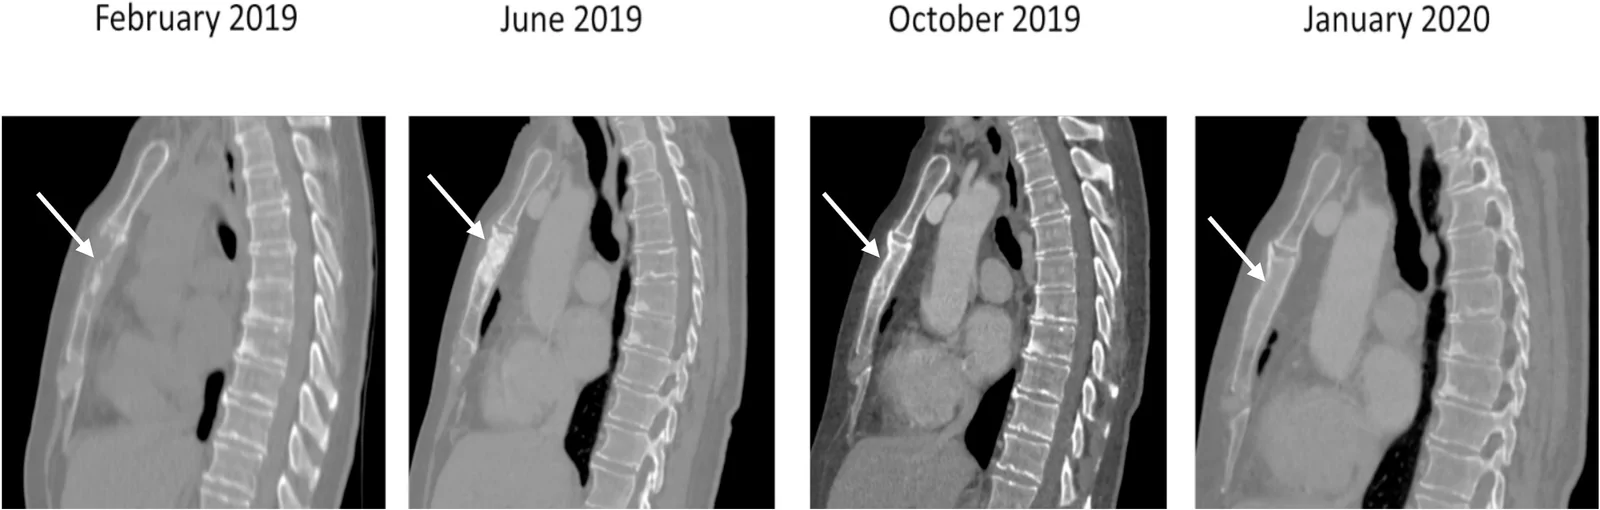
Tumor response of sternal bone metastases under immunotherapy and denosumab: serial sagittal CT scans from February 2019 to January 2020.
